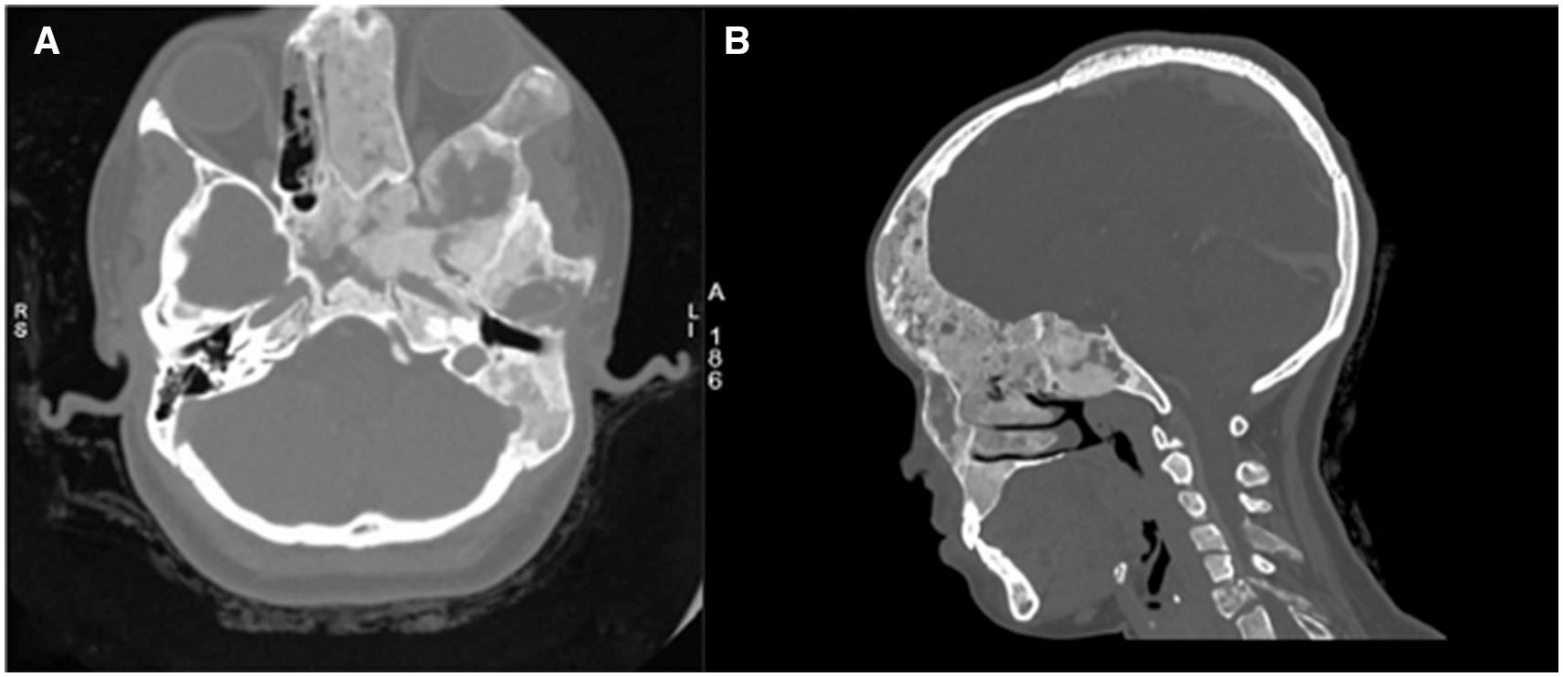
(A) CT cranium axial bone window showing bone expansion with ground glass matrix in left ethmoid sinus, sphenoid bone with narrowing of left optic canal and petrous and mastoid portion of left temporal bone. (B) CT cranium with cervical spine sagittal plane bone window showing ground glass matrix in left frontal bone, maxilla, C4 spinous process, and C6 cervical vertebral body.
